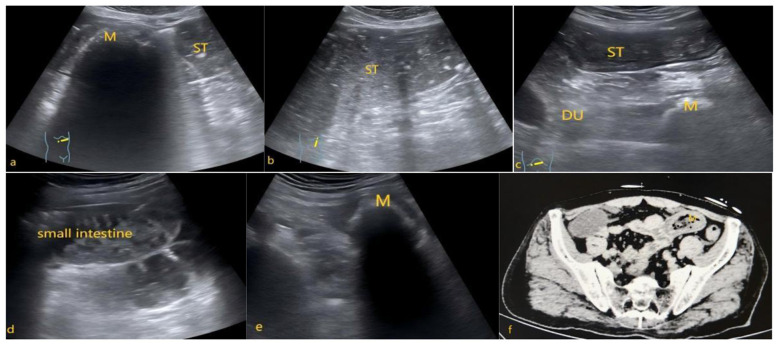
Ultrasonic characteristics of bezoars. Case 1: (a) Phytobezoar impacted in the pylorus; (b) Massive retention of gastric contents. Case 2: (c) Phytobezoar impacted in the horizontal part of the duodenum; (d) Later rechecking revealed jejunum obstruction; (e) A mass of bezoar was visible, followed by acoustic shadowing, impacted in the distal jejunum cavity; (f) CT also shows a gas-filled mass of bezoar in the left mid-abdominal jejunum. M, phytobezoar; ST, stomach; DU, duodenum.
